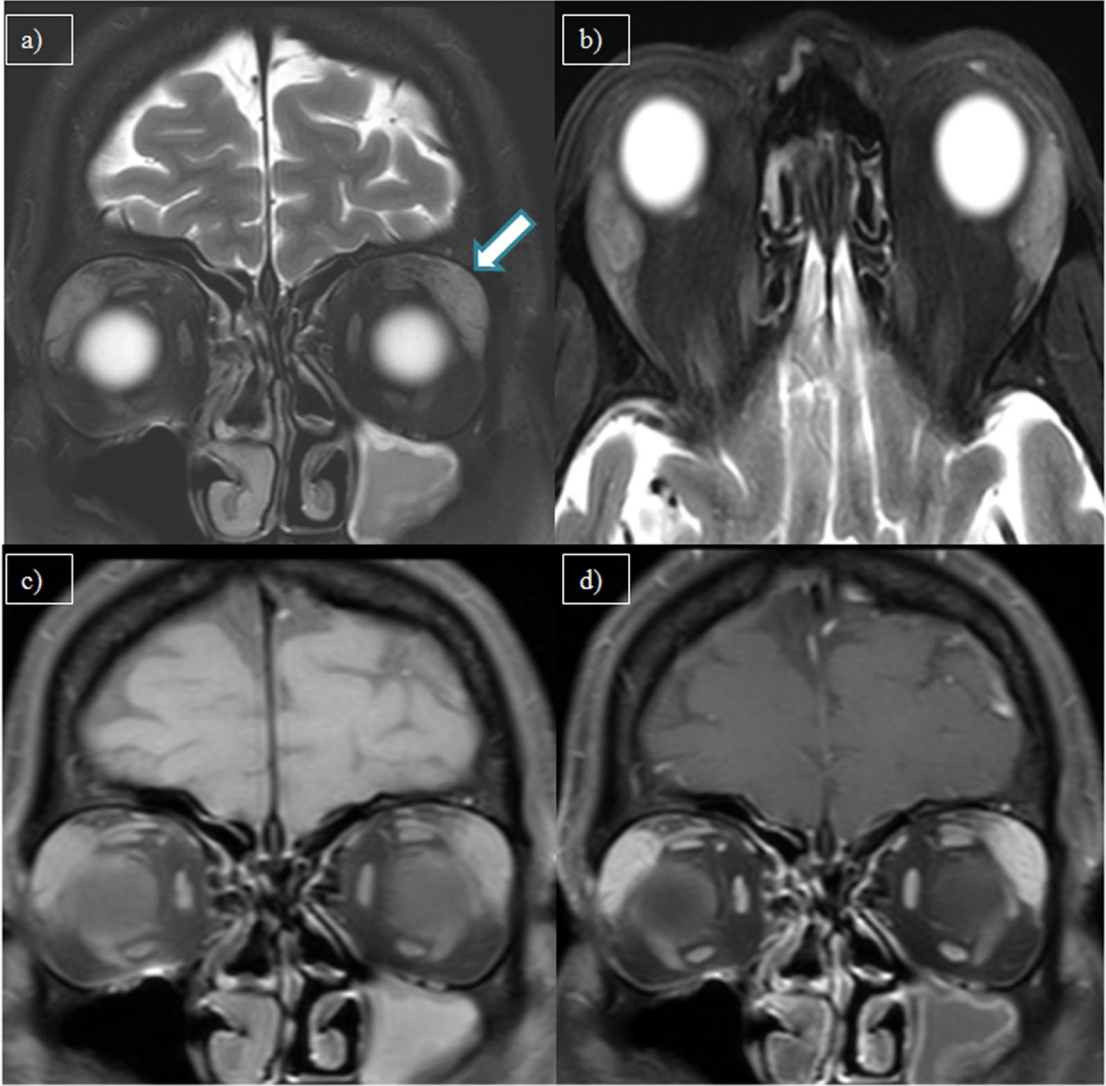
a MRI coronal T2W-FS, b axial T2W-FS, c coronal pre-contrast T1W-FS, and d coronal post-contrast T1W-FS images of a 49-year-old male patient with complaints of headache showing bilateral symmetrical enlargement of lacrimal gland appearing hyperintense on T2W (arrow) and showing homogenous post-contrast enhancement—dacryoadenitis
